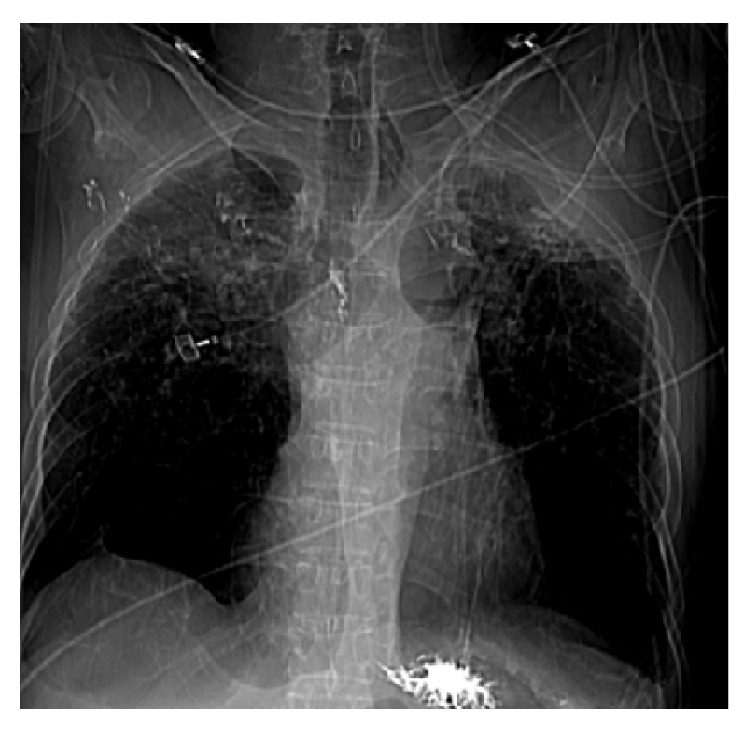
Chest X-ray (CXR) showed extensive fibrocalcific scarring in the upper lobes and compensatory emphysema of the lower lobes.
